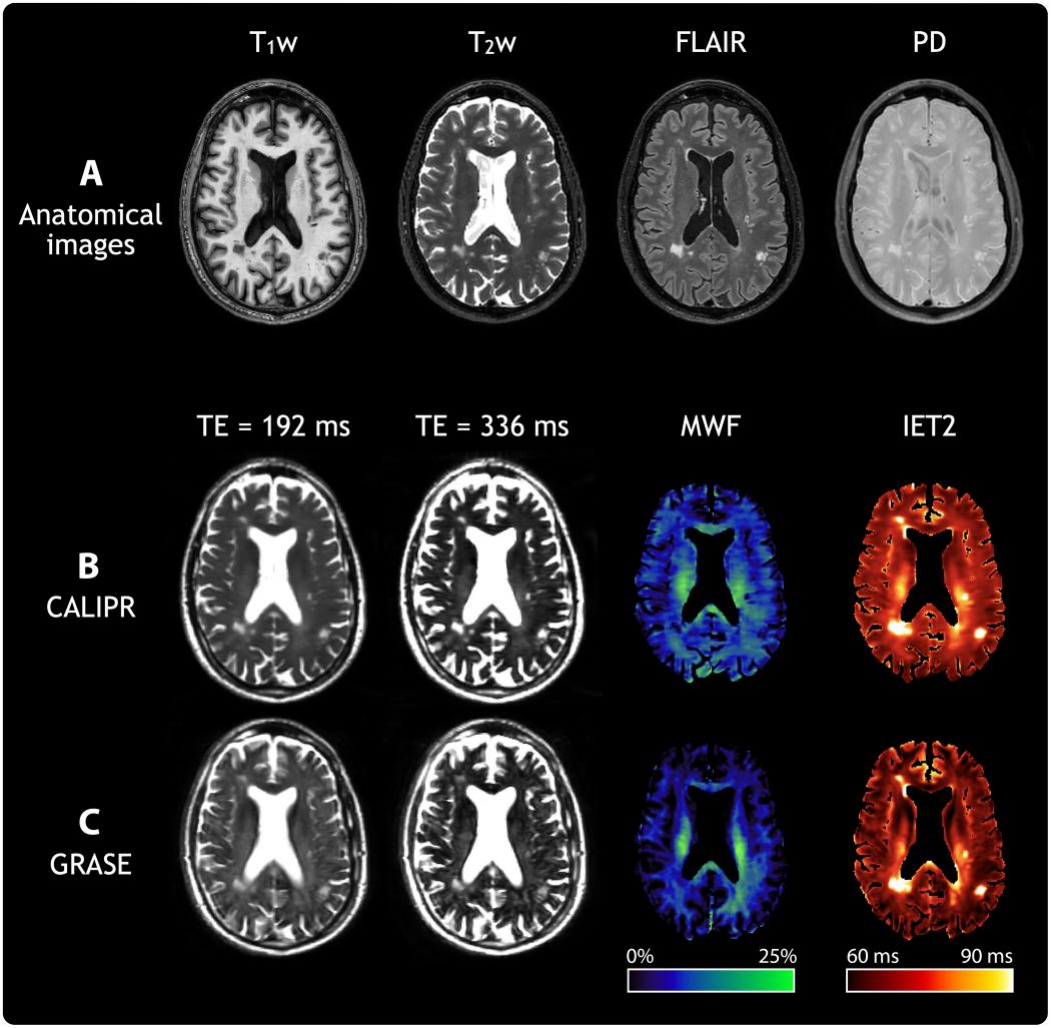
Anatomical and MWI results for a subject living with multiple sclerosis. A representative image slice from anatomical and MWI results in brain for a subject living with relapsing-remitting multiple sclerosis (male, age 60 years, expanded disability status scale of 2.0, and disease duration of 12 years). (A) From left to right, the columns show T_1_-weighted (T_1_w), T_2_w, fluid-attenuated inversion recovery (FLAIR), and proton density (PD) anatomical images. For (B), our proposed CALIPR MWI approach and (C) a commonly used GRASE MWI approach, from left to right the columns, show two MWI images at different echo times (TE) as well as quantitative MWF and geometric mean of IET2 maps. The same slice is shown for all images and maps, which were aligned in the subject’s T_1_w image space.
